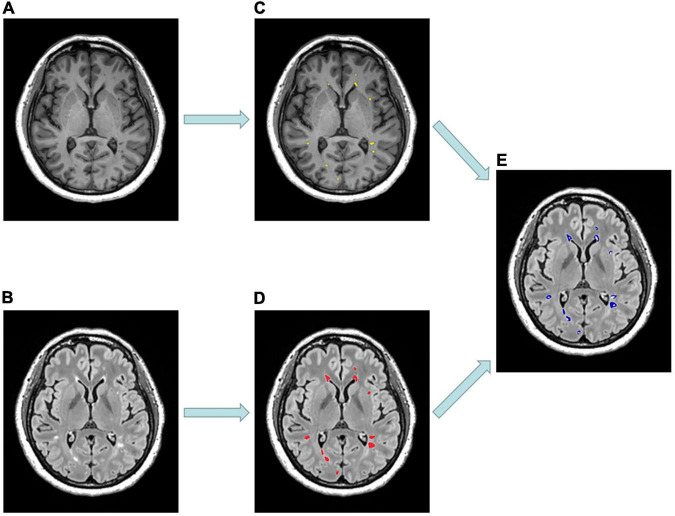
Example of MR scans and lesion masks of a 53-year-old patient diagnosed with relapsing-remitting multiple sclerosis. (A) Selected axial MPRAGE image with hypointense lesions. (B) Selected axial FLAIR image with hyperintense lesions. (C) T1-hypointense lesions (T1Ls) mask (yellow). (D) T2-hyperintense lesions (T2Ls) mask (red). (E) Pure T2-hyperintense lesions (pure-T2Ls) mask (blue) was obtained by subtracting (C) from (D).
